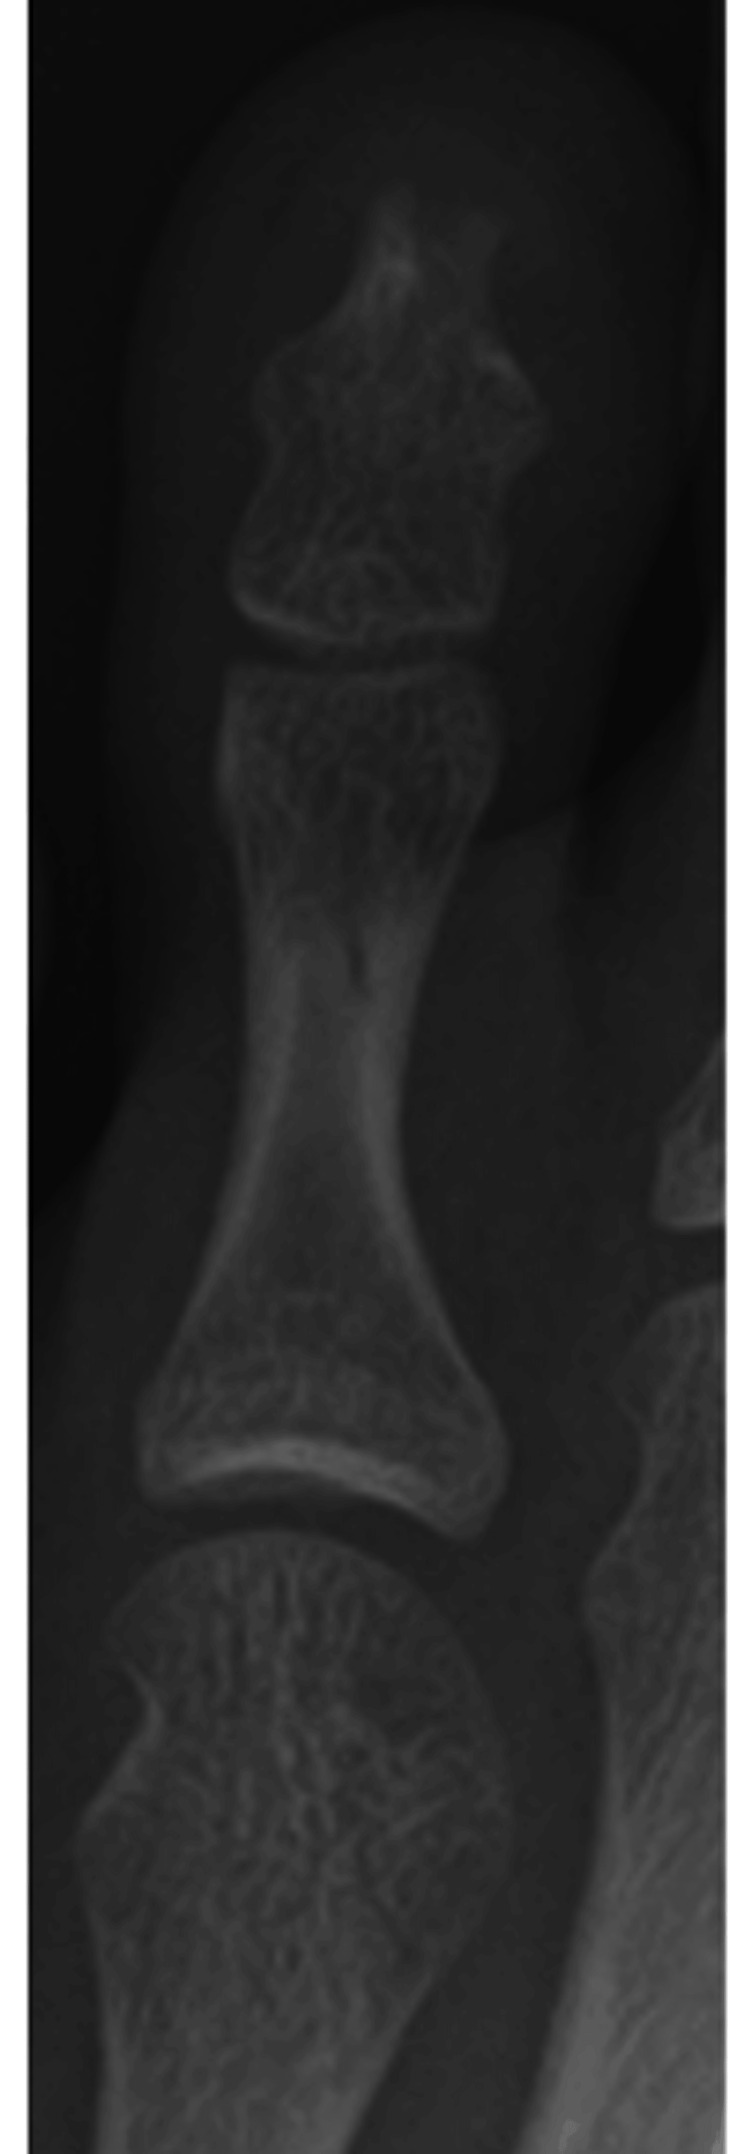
X-ray six months after revision surgery.
